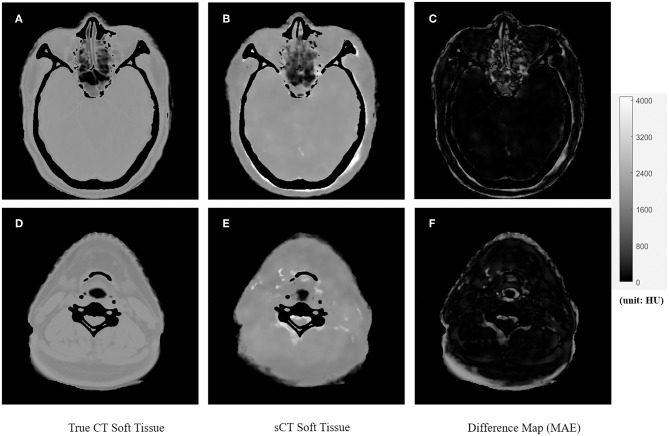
The comparison of true CT and synthetic CT for soft tissues. The first column is true CT images. The second column is the synthetic CT images. The third column is the difference maps. (A–C) Showed soft tissue in the head and nasal region. (D–F) Showed soft tissues in the neck region. Gray bar indicated the mapping from CT number to gray scale in the difference maps.
